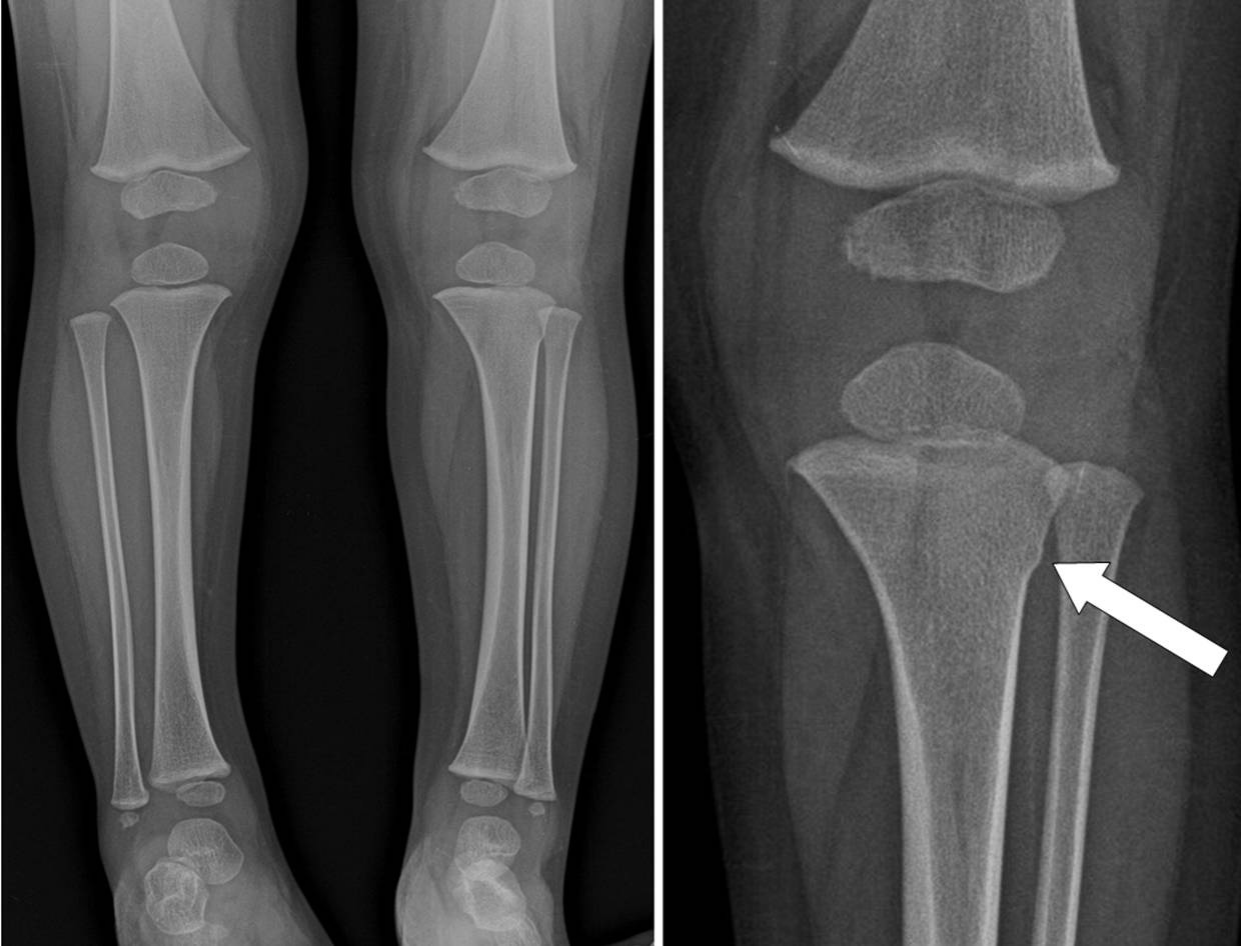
Whole lower extremity radiograph showed a metaphyseal torus fracture of the left tibia (*arrow*).
